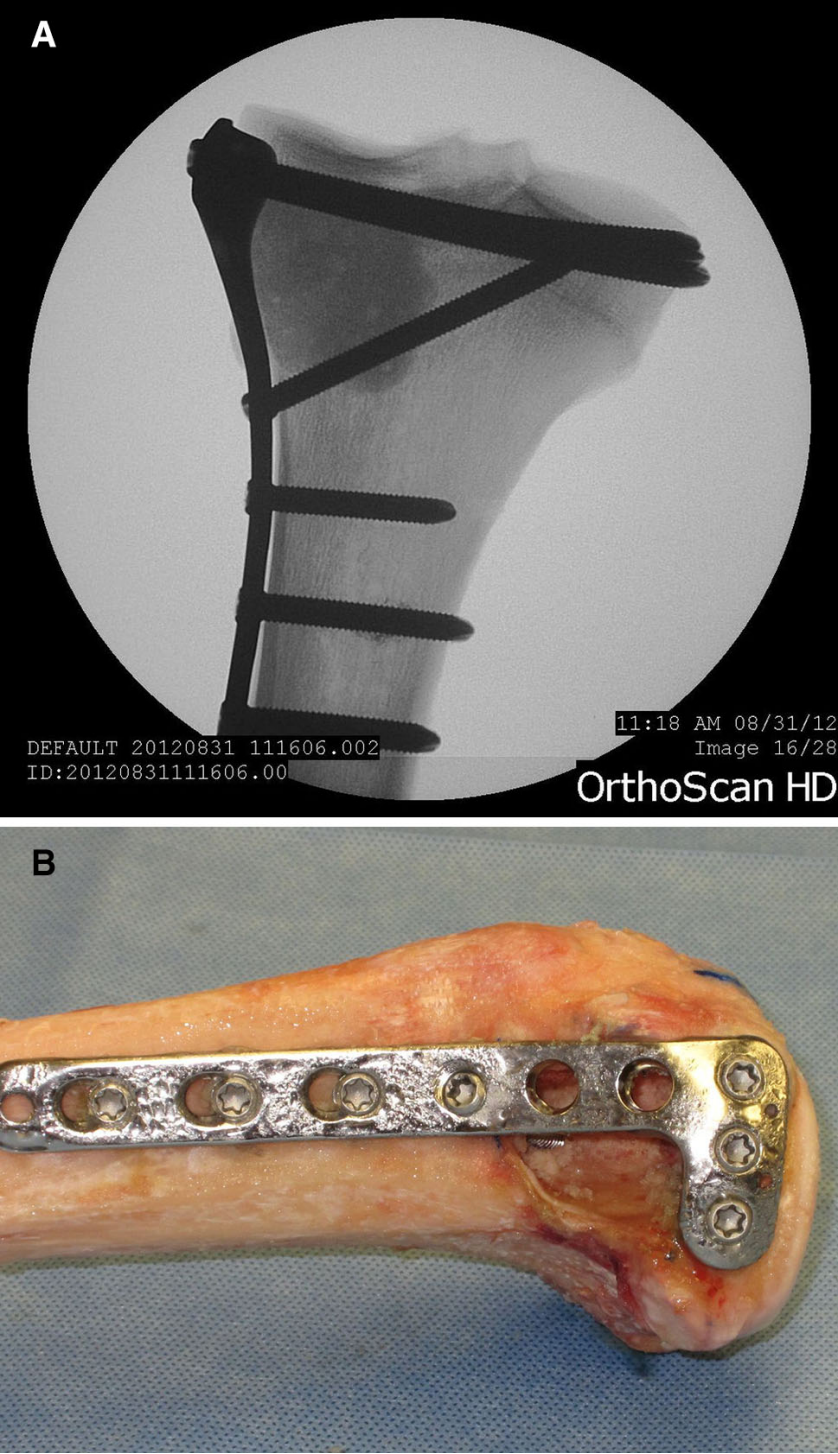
Radiograph after PMMA (a) and gross image before PMMA (b) of reconstruction with a locking plate
